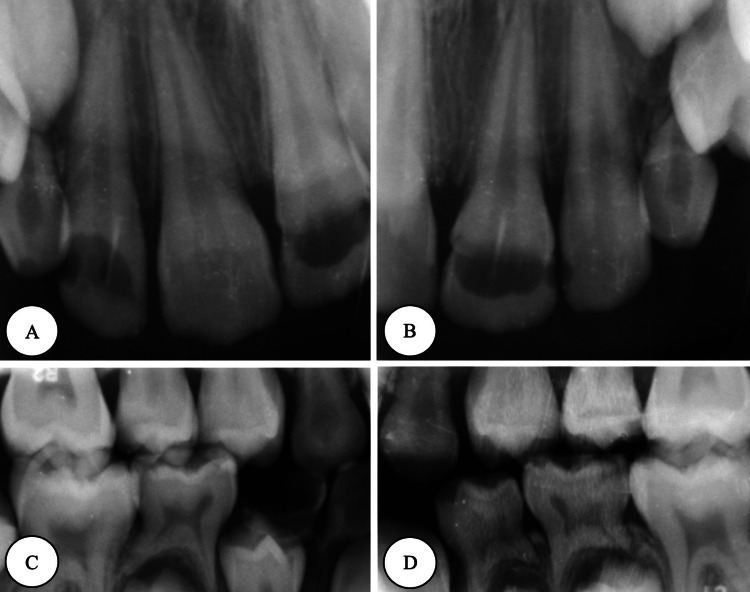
Preoperative radiographs (A) Periapical right-shifted radiograph. (B) Periapical left-shifted radiograph. (C) Right-side bitewing. (D) Left-side bitewing
